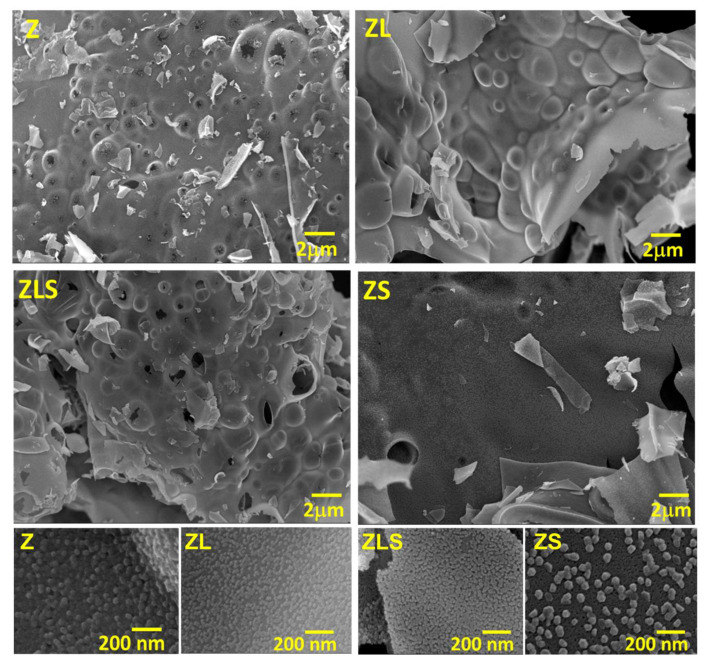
SEM observation of Z, ZL, ZLS, and ZS and the effect of the ultrasound-assisted synthesis route in their microstructure at low and high magnification.
